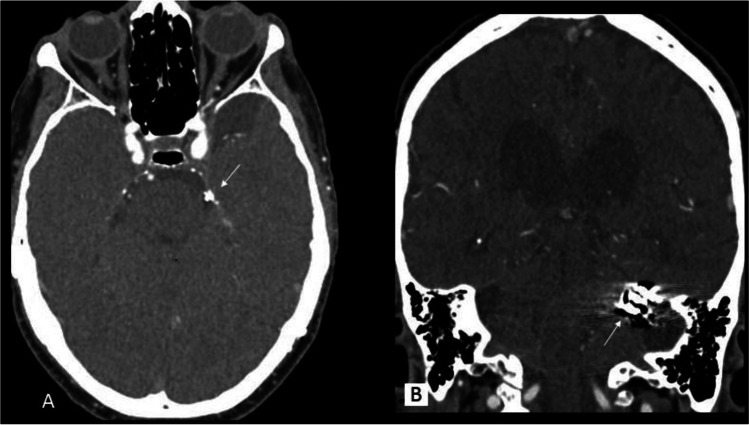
Postoperative angio-CT scan showed the clip (arrow) of the DAVF in position and disappearance of the fistulous connection. The brainstem and left mesial temporal lobe regained their normal positions in the middle incisural space and middle fossa, respectively (axial and contrast enhanced coronal CT images). MRI was not performed due to possible clip artifacts
